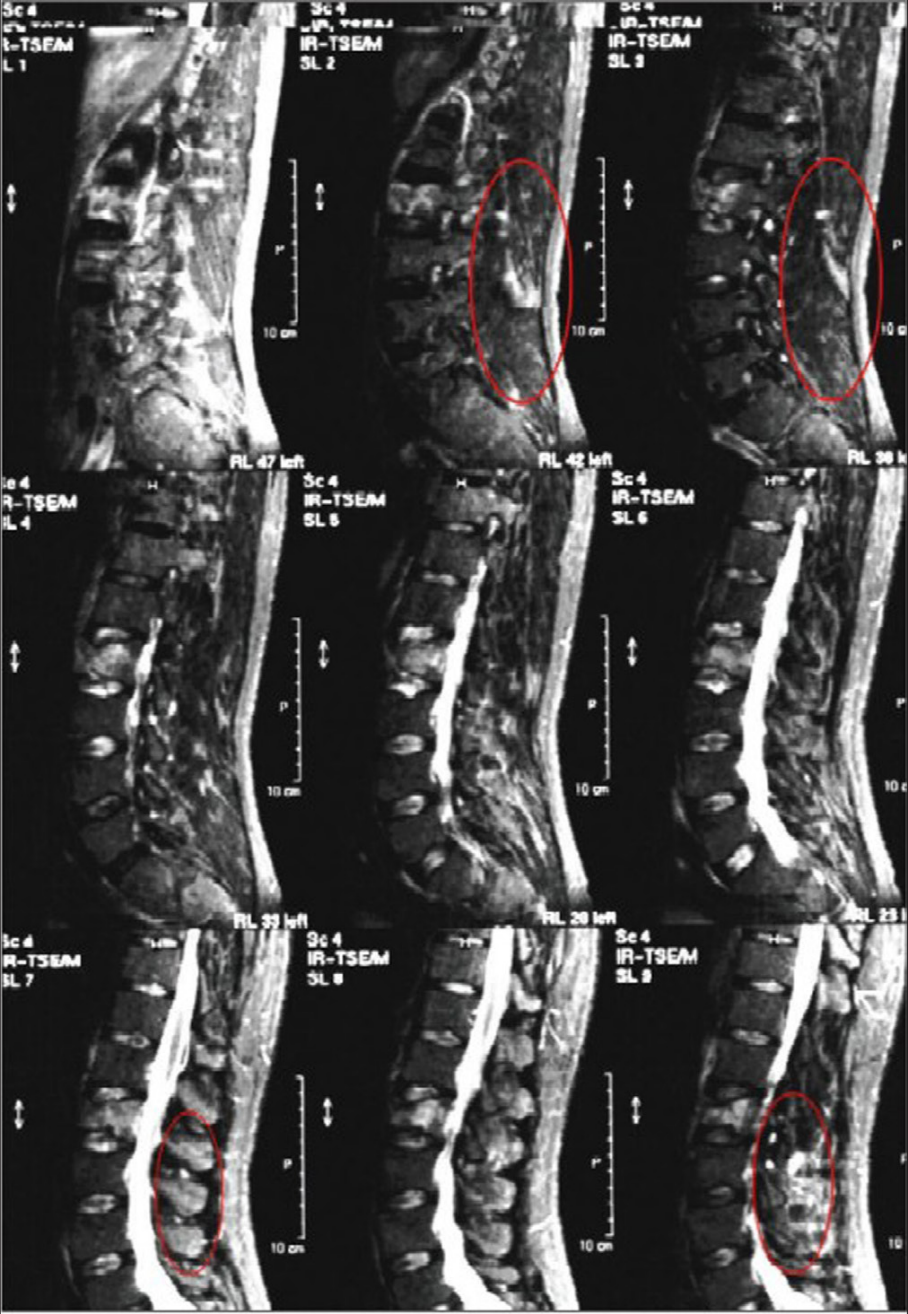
MRI-STIR (MRI-short tau inversion recovery) study shows hyper-intense signal in the interspinous ligaments suggestive of ligamentous damage
